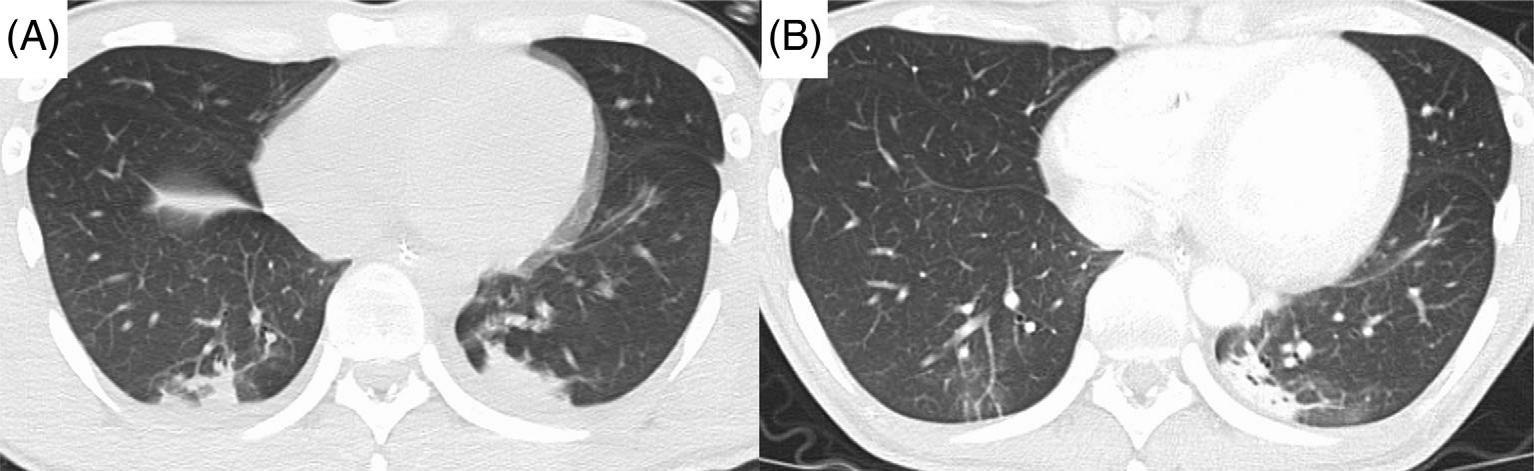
Chest computed tomography of case 1 before (A) and after (B) mechanical insufflation‐exsufflation
